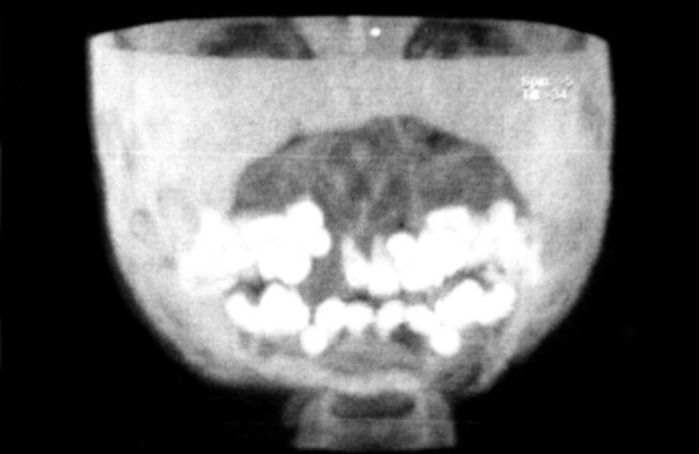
CT-image showing floating teeth
